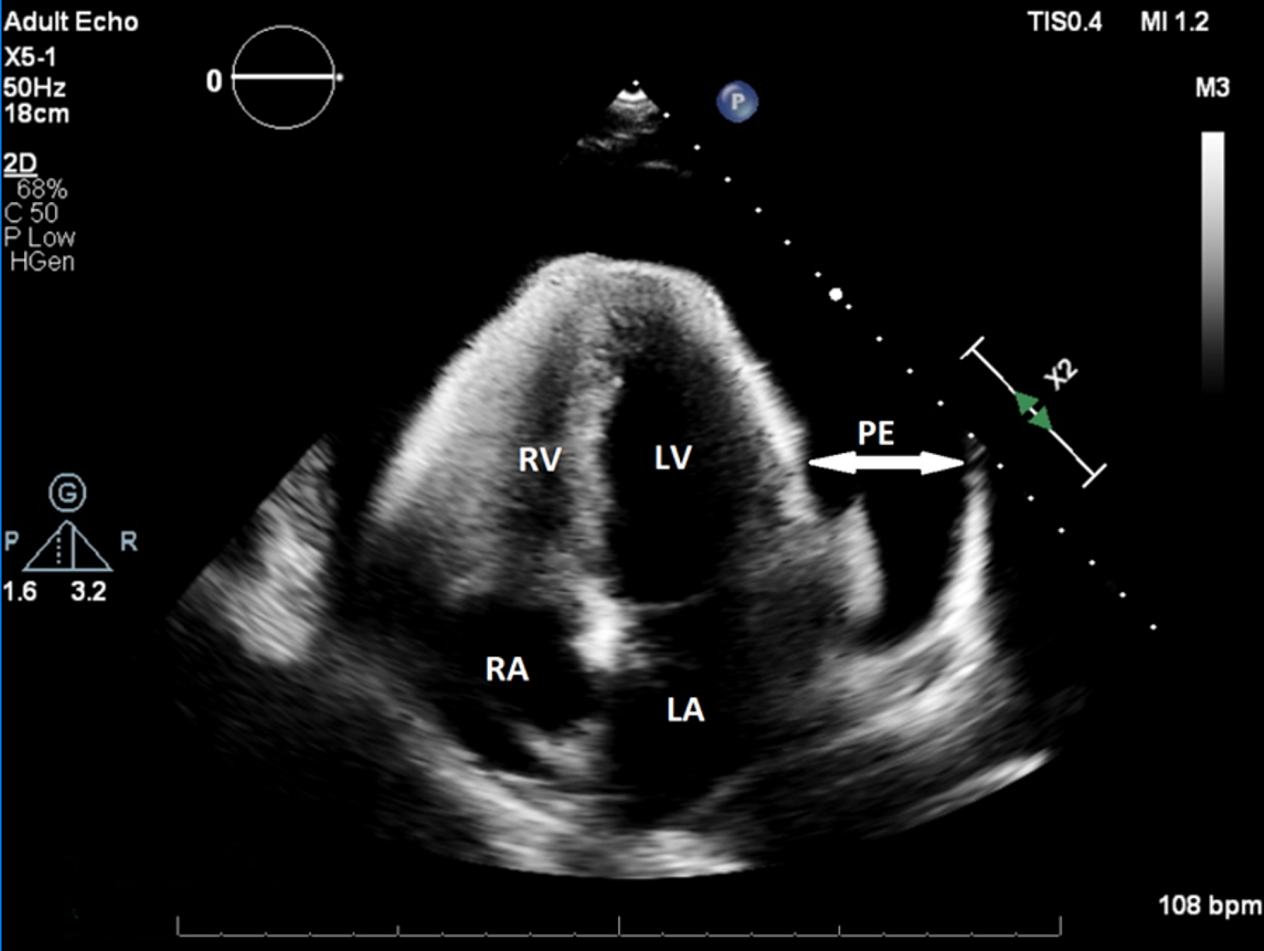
Apical four-chamber view of the 2D transthoracic echocardiogram revealing a large pericardial effusion LA - left atrium; RA - right atrium; LV - left ventricle; RV - right ventricle; PE - pericardial effusion
